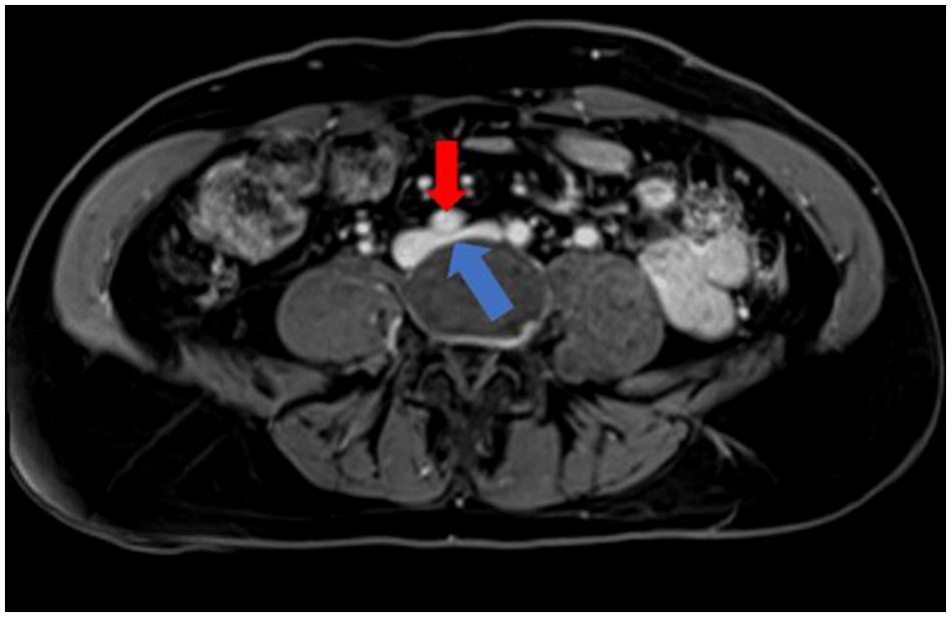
Axial cross-sectional magnetic resonance image demonstrating severe compression of the left common iliac vein (LCIV) (blue arrow) between the right common iliac artery (RCIA) (red arrow) anteriorly and the spine posteriorly. The anteroposterior diameter of the LCIV is narrowed by more than 50% at the compression point when compared with the adjacent venous segment.
